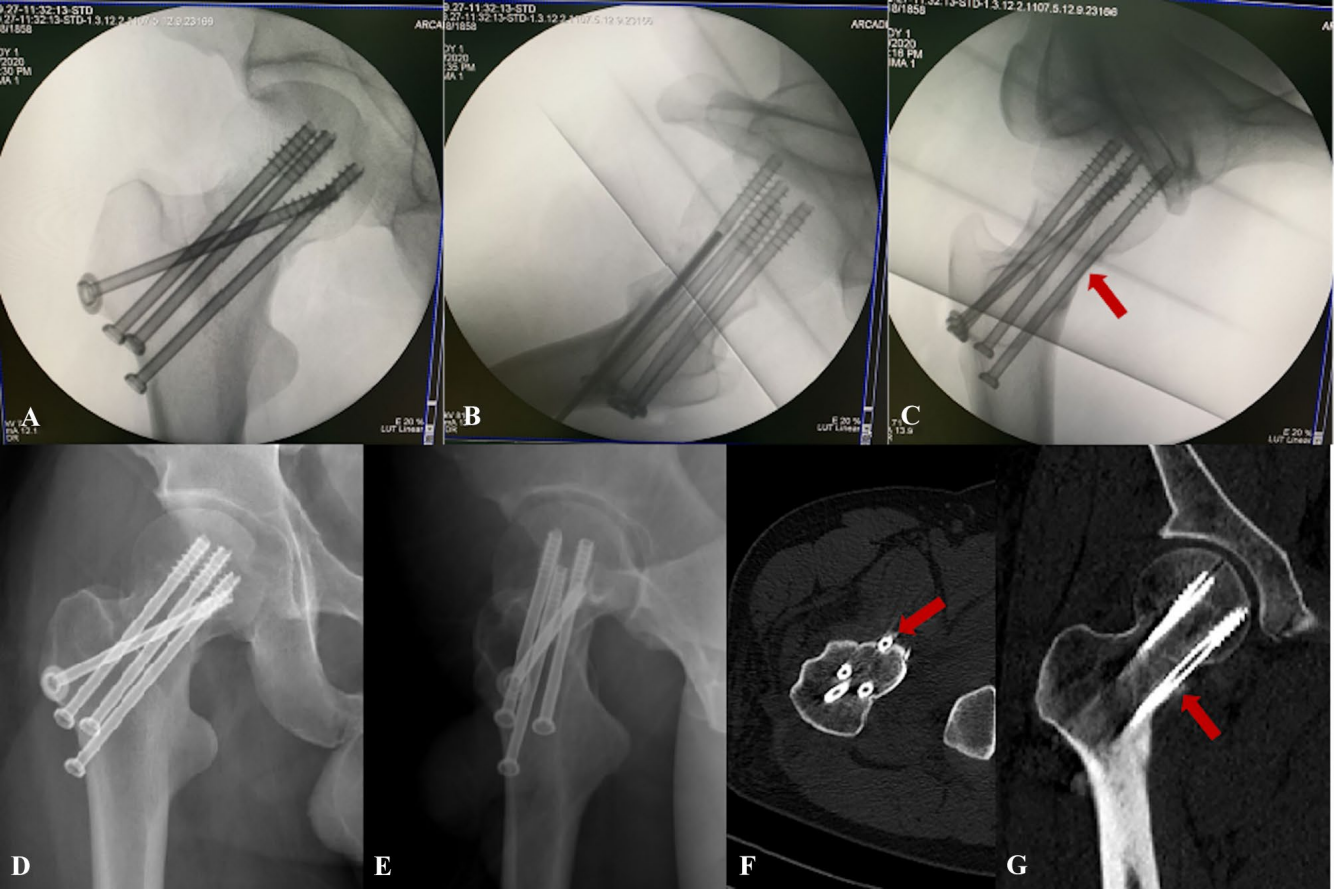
(A,B) Intra-operative the AP and lateral views showed all screws were contained. (C) Intra-operative the tangential view showed the inferior screw IOI. (D,E) Postoperative plain radiographs showed all screws were contained. (F,G) Postoperative CT showed the inferior screw IOI. The red arrow represents the inferior screw IOI.
